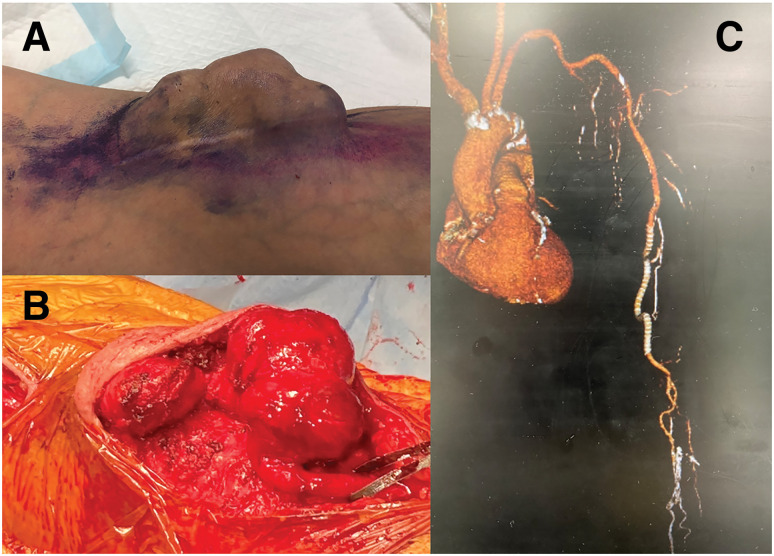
(A) Large, painful SBA aneurysm. (B) Intraoperative view showing a large mass at the puncture site. (C) Contrast CT after graft replacement. SBA: superficialized brachial artery; CT: computed tomography
